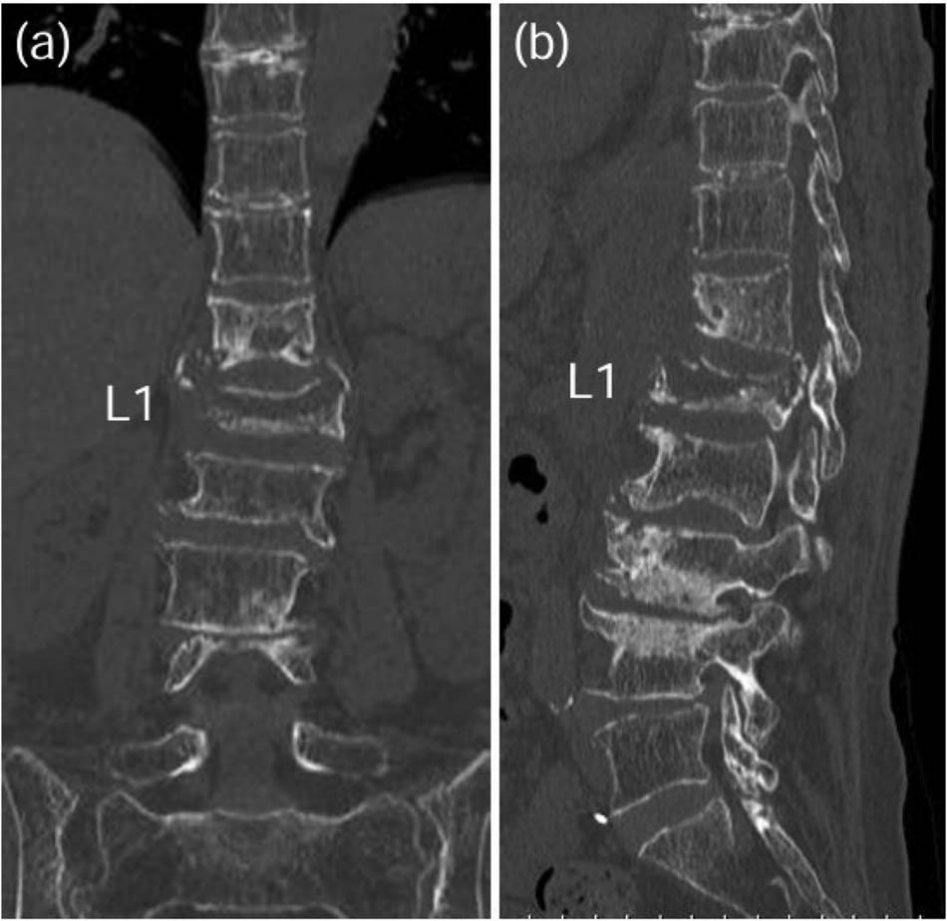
CT scan revealed osteolysis in the L1 vertebral body and the inferior endplate of Th12 [coronal view: (a), sagittal view: (b)].
